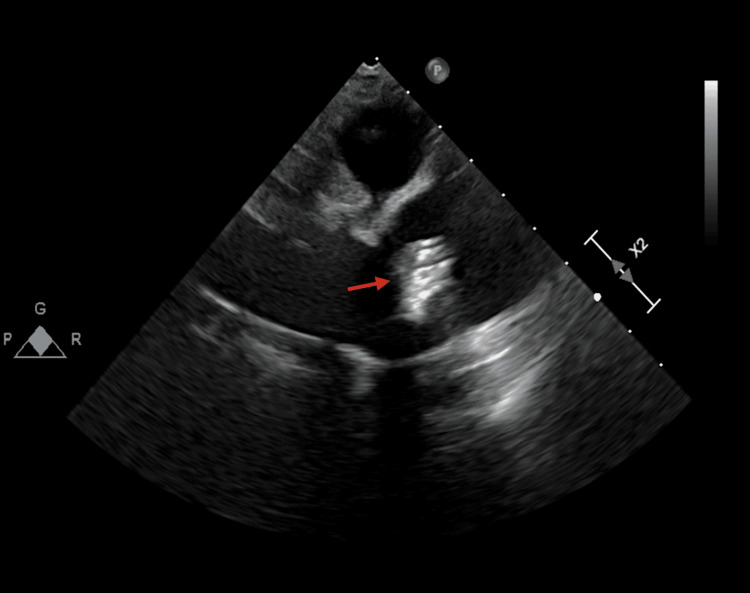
Transesophageal Echocardiography Image Showing the Mobile Mass Transesophageal echocardiography image demonstrating a pedunculated, mobile echogenic mass at the distal anastomotic region of the ascending aortic graft (red arrow). The mechanical aortic valve prosthesis is visualized and appears well seated.
